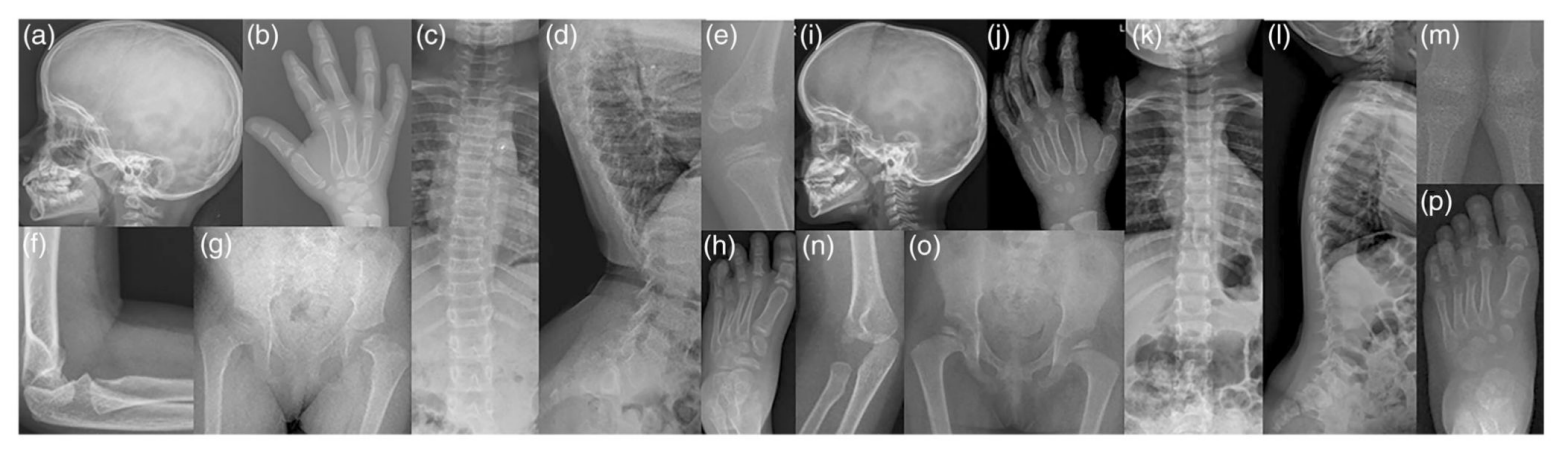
Radiographs of Proband 1 (a−h) at age 8 years and Proband 2 (i−o) at the age of 2 years revealed copper beaten appearance of skull (a, i), clinodactyly of fifth finger, shortened second proximal phalanx, shortened third and fourth metacarpals with small carpals (b) small carpal bones with delayed ossification (j), mild scoliosis (c), and lack of increase in lumbar interpedicular distance (k), lumbar lordosis is evident in both (d, l), along with platyspondyly with anterior beaking of vertebrae (d), small epiphysis, metaphyseal sclerosis, cupping, and fraying of metaphysis at knee joint are exhibited in both (e, m), subluxation of elbow joint (f) and elbow joint dislocation (n), short femoral neck (g, o) with coxa breava (o) and short fourth metatarsal bone is observed in both (h, and p) and short third metatarsal bone (p).
